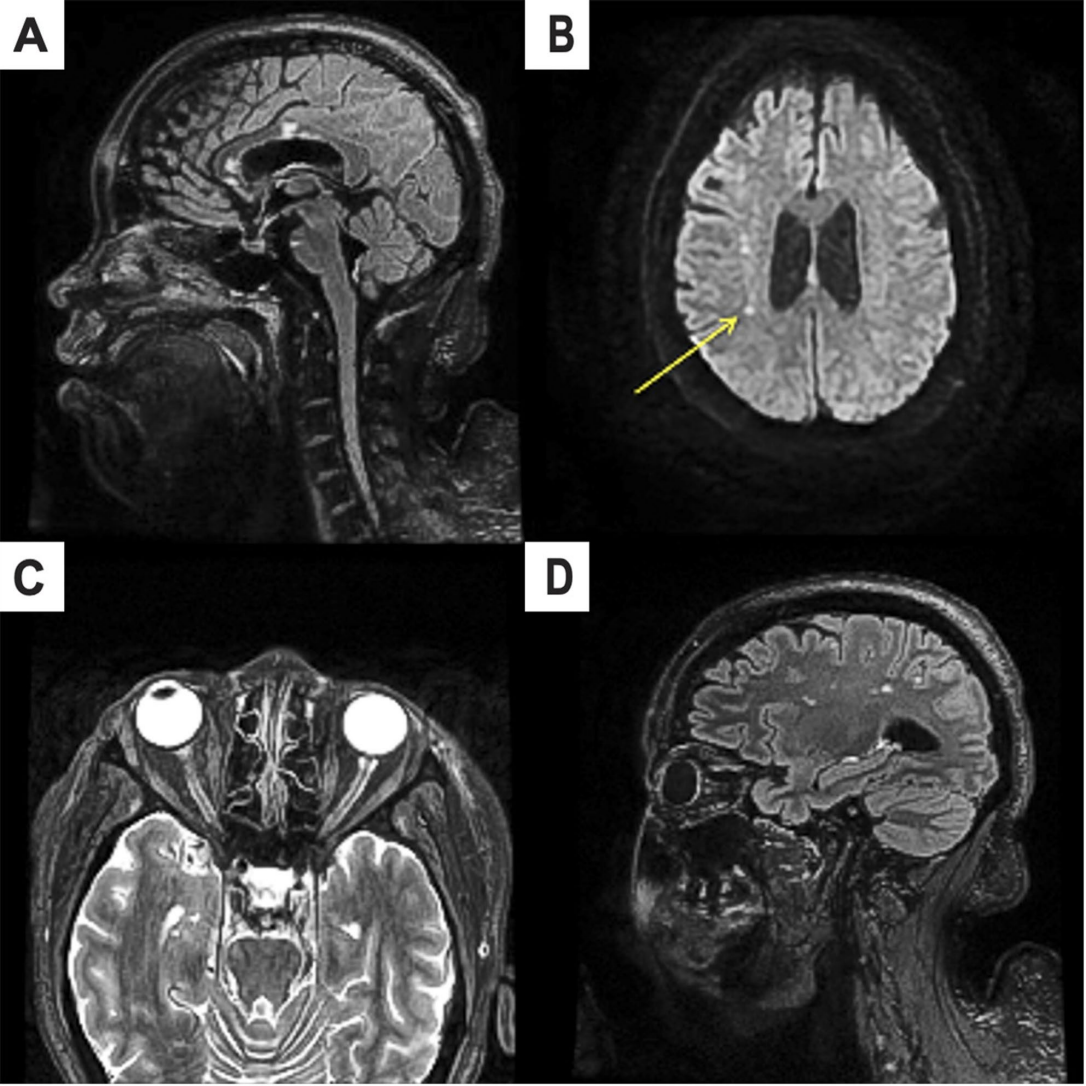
MRI brain and orbits with and without contrast findings. (A) T2 fluid-attenuated inversion recovery (FLAIR) hyperintensities along the right genu and body of the corpus callosum and ventral pons. (B) Diffusion restriction involving right corona radiata (C) MRI orbit with and without contrast demonstrating bilateral optic atrophy. (D) T2 FLAIR sagittal view redemonstrating lesions within right corona radiata
